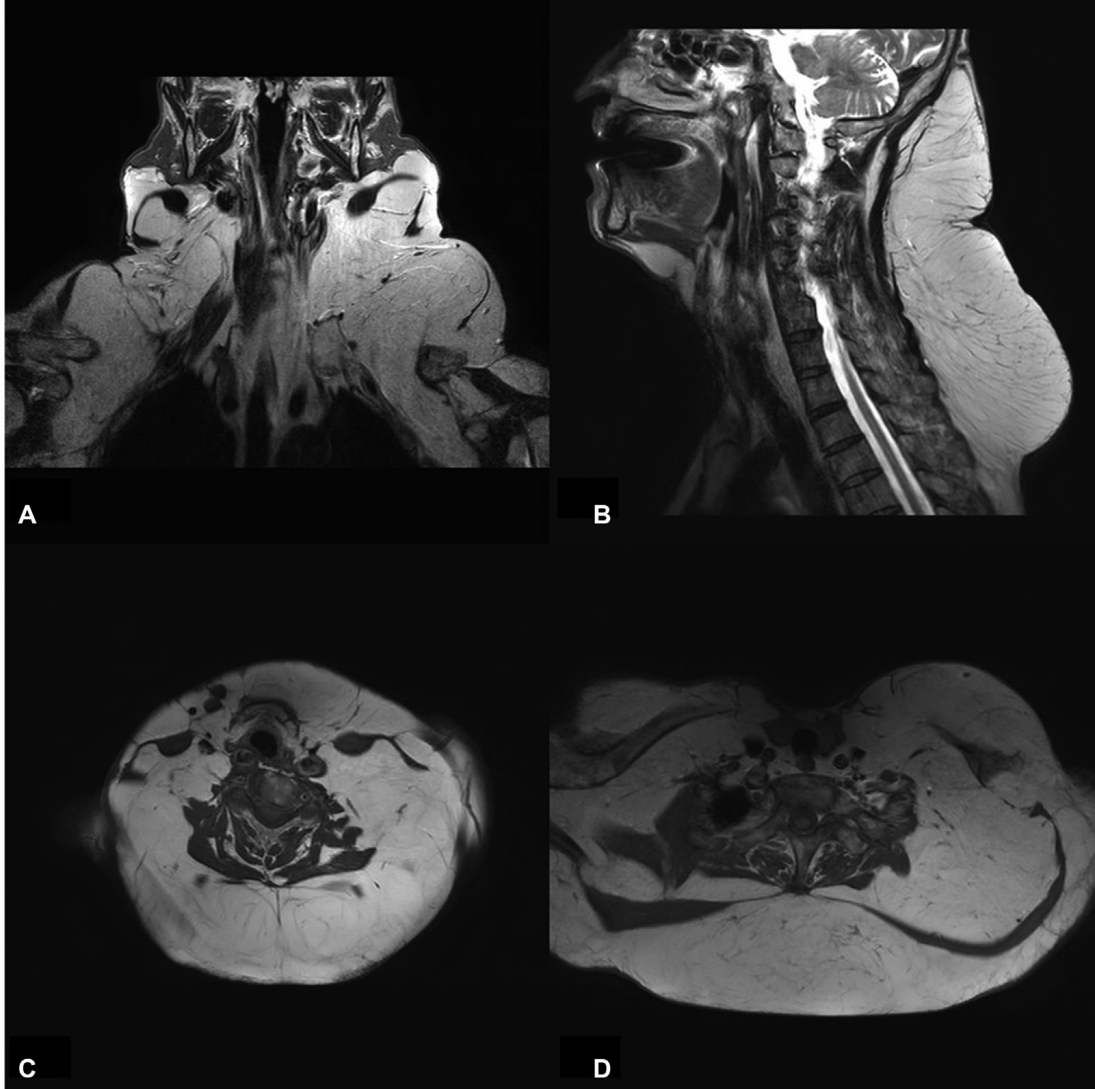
Preoperative magnetic resonance imaging (MRI) images. Preoperative MRI revealed hypertrophic fat tissue around the neck and anterior chest wall, which was consistent with Madelung's disease (
